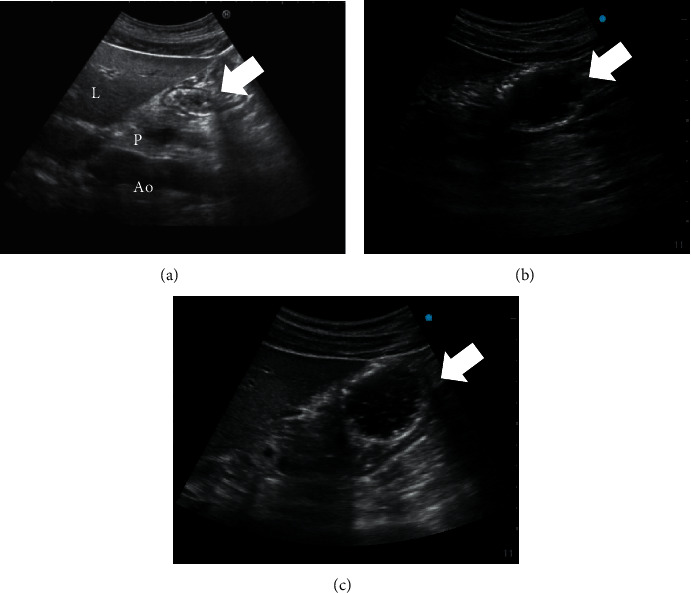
(a) Sagittal sonogram of the empty gastric antrum. The antrum is located between the left lobe of the liver and the pancreas at the level of the aorta or inferior vena cava. (b) Sagittal sonogram of the gastric antrum 10 minutes after the ingestion of 150 ml of diluted whisky (30 ml of whisky + 120 ml of water). The antrum appeared distended with a hypoechoic fluid content. (c) Sagittal sonogram of the gastric antrum 10 minutes after the ingestion of 150 ml of 12.5% glucose solution. The antrum appeared distended with hypoechoic fluid. Arrow, antrum; L, liver; P, pancreas; Ao, aorta.
